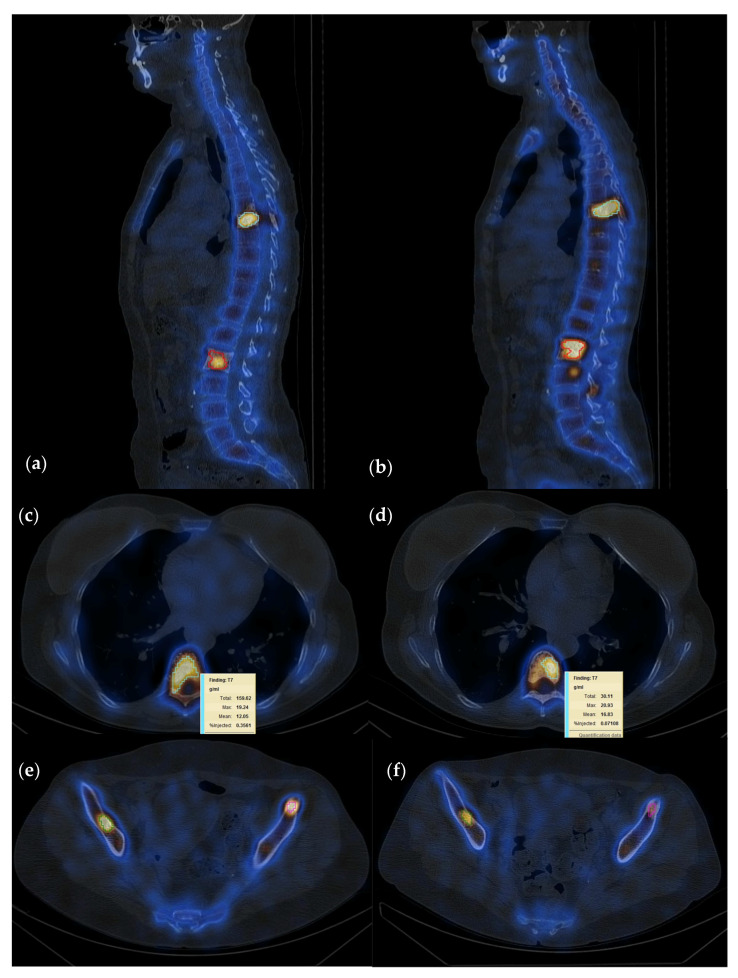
Fifty-five-year-old female patient with invasive lobular carcinoma operated in 2008 (left mastectomy) treated with chemotherapy, radiotherapy and hormone therapy; local recurrence and bone metastasis diagnosed in October 2021; multiple metastatic lesions with high uptake localized at T7, L2, and both iliac spines currently in treatment with osteoclast inhibitors; (a–c) baseline study; (d–f) follow-up study after 6 months of osteoclast inhibitor; quantification showed a summed SUVmax 81.3 g/mL on the baseline and 47.2 g/mL on the follow-up study, suggesting PR with a decrease in radiotracer uptake of 58%.
